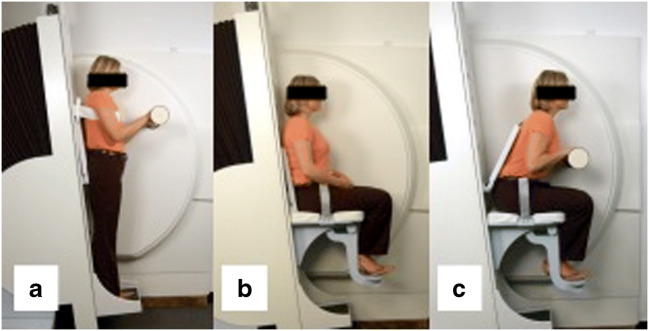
Upright MRI scanner enables the examination of the patient in different body positions. In the present study, patients were scanned in positions a, b and c during a single examination
